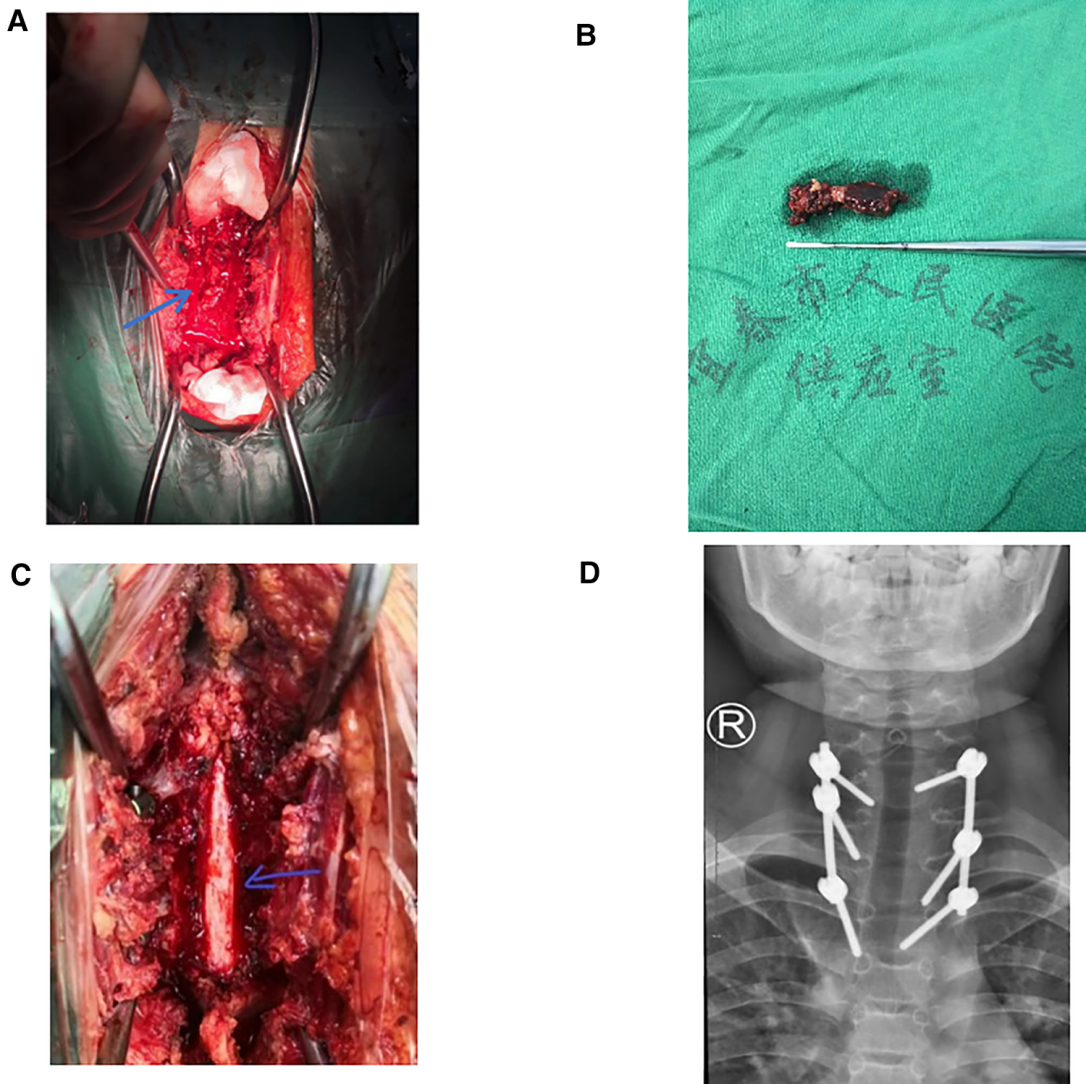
(A) Severe dural sac compression before surgery, (B) intraoperative excision of the tumor, about 5 cm long, (C) the dural sac was entire and pulsating after tumor resection, (D) postoperative radiographs showed accurate pedicle screw placement.
